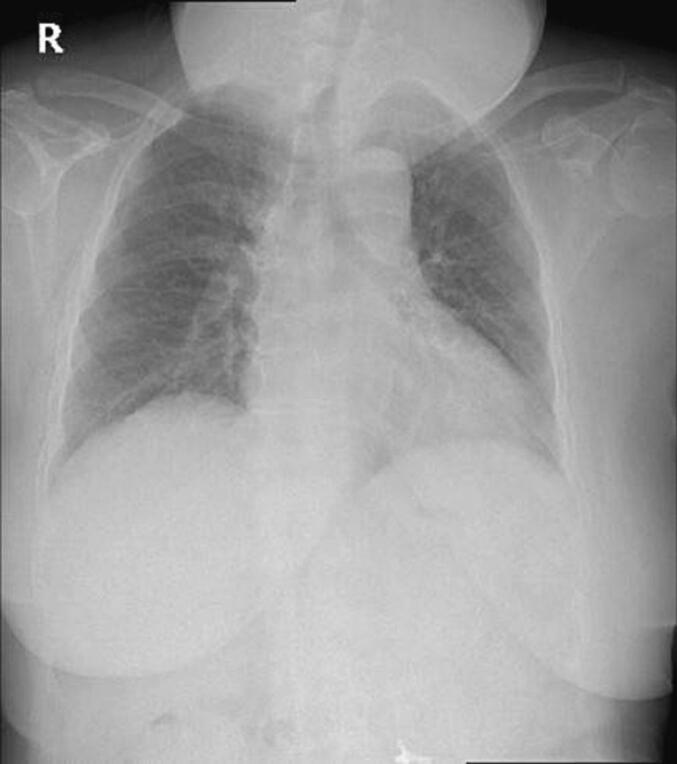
Chest X-ray examination.
